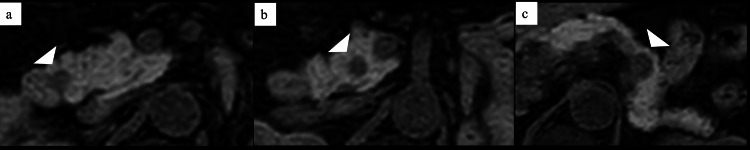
T1-weighted MRI with fat suppression T1-weighted MRI with fat suppression reveals two distinct low-signal nodules in the pancreatic head (a, b; arrowhead) and one in the pancreatic tail (c; arrowhead). These nodules were subsequently confirmed to be metastatic lesions from RCC. RCC, renal cell carcinoma
